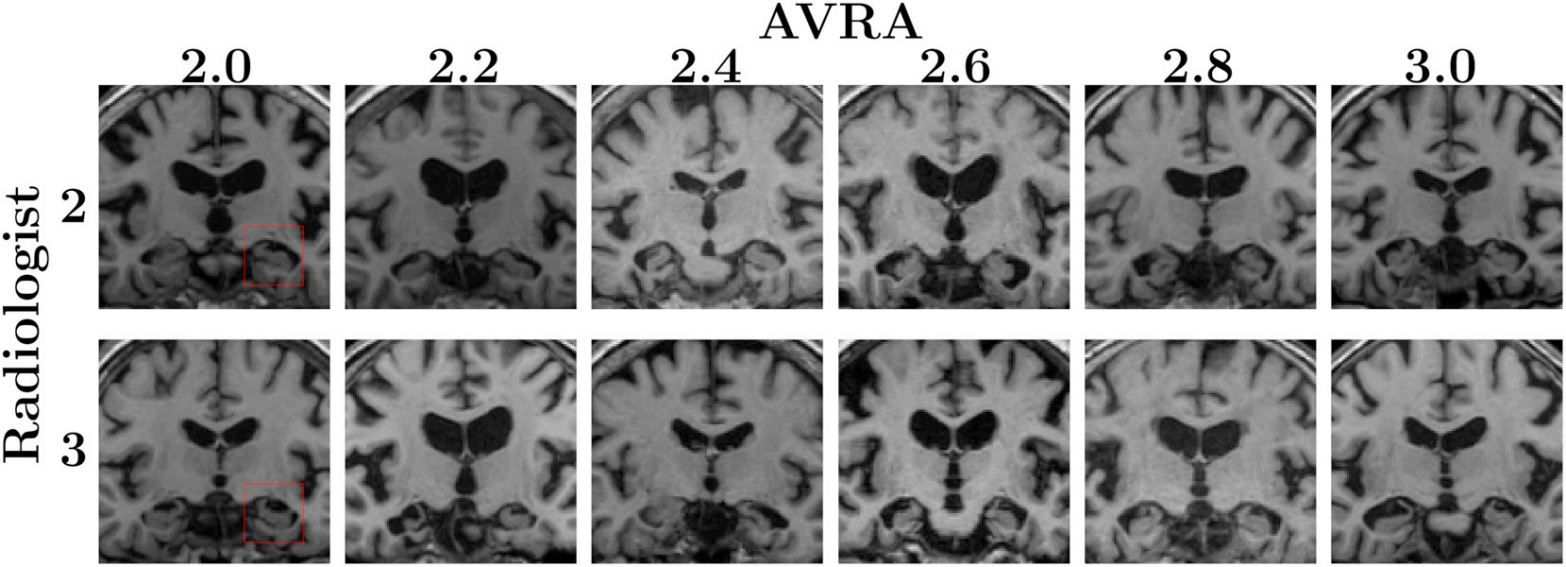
Comparison between AVRA's continuous ratings and the neuroradiologist's discrete ratings of the same images. *Rows*: MRI slices with MTA on the right side of the image (side indicated by the red squares) rating of 2 (top) and 3 (bottom) given by neuroradiologist. *Columns*: corresponding continuous AVRA ratings. E.g., the second image from the left in the bottom row was given assessed to have a left MTA score of 3 by the neuroradiologist and 2.2 by AVRA. When the radiologist re-examined these cases the same ratings were given for all images, except for the three images on the right in the top row (Radiologist: 2, AVRA: 2.6, 2.8 and 3.0), which were instead given MTA scores of 3. The image rated 2 by L.C. and 2.4 by AVRA was described as a subject between 2 and 3.
